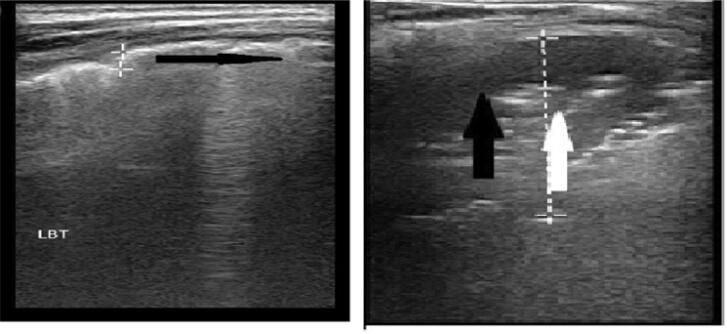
Normal and abnormal lung ultrasound findings.
